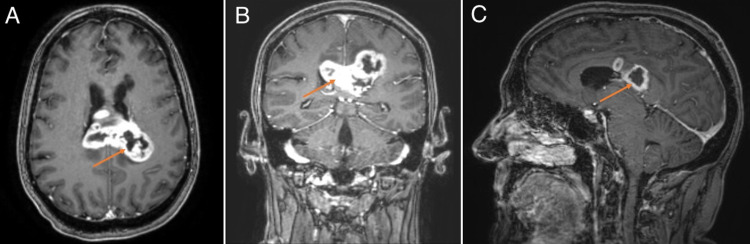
MRI brain demonstrating a heterogeneously enhancing mass with cystic and necrotic changes centered within the splenium of the corpus callosum. (A), (B), and (C) are T1-weighted post-contrast axial, coronal, and sagittal views, respectively.
